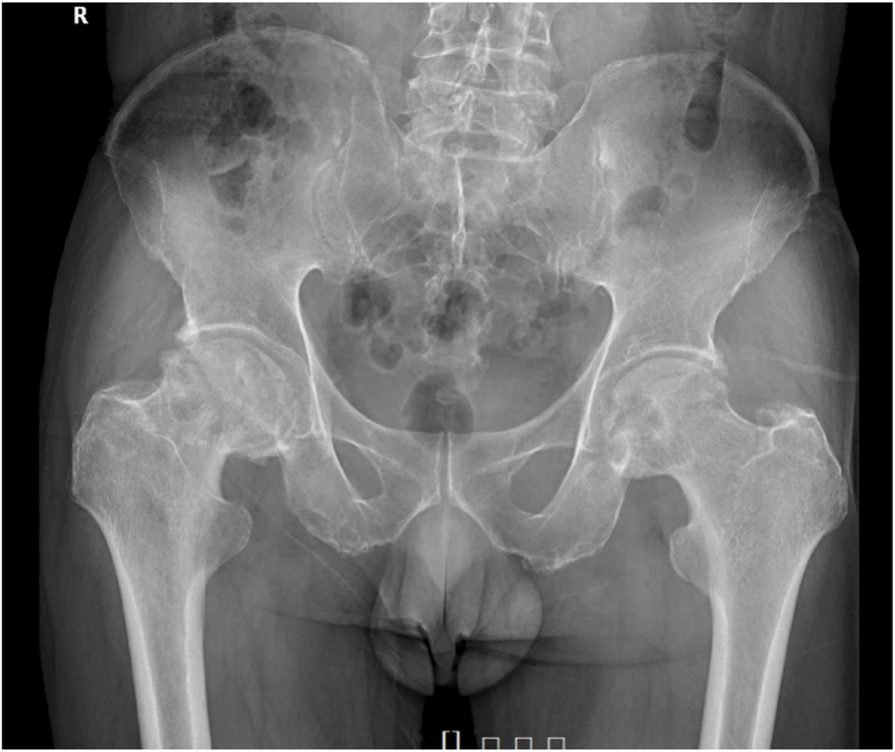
Plain radiograph of pelvis before right hip arthroplasty. Bilateral Ficat IV stage avascular necrosis of femoral head is shown
